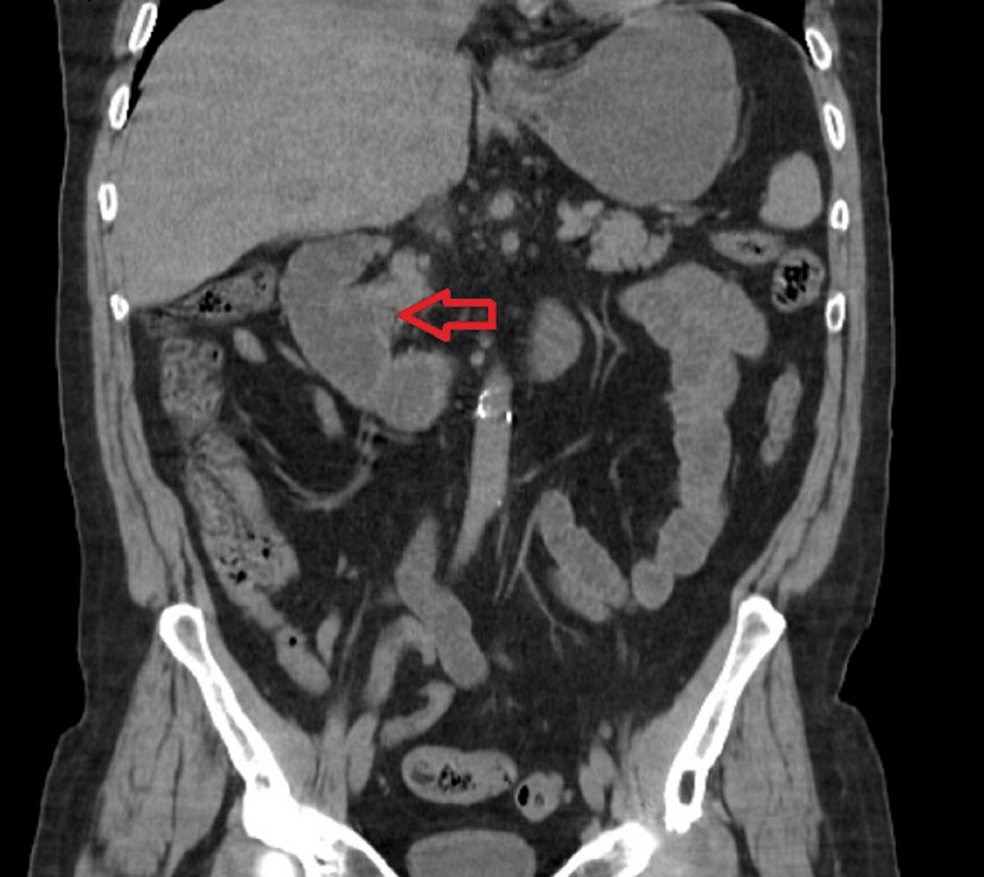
CT of the abdomen and pelvis without contrast showed a diverticulum at the ampulla of Vater (red arrow).
